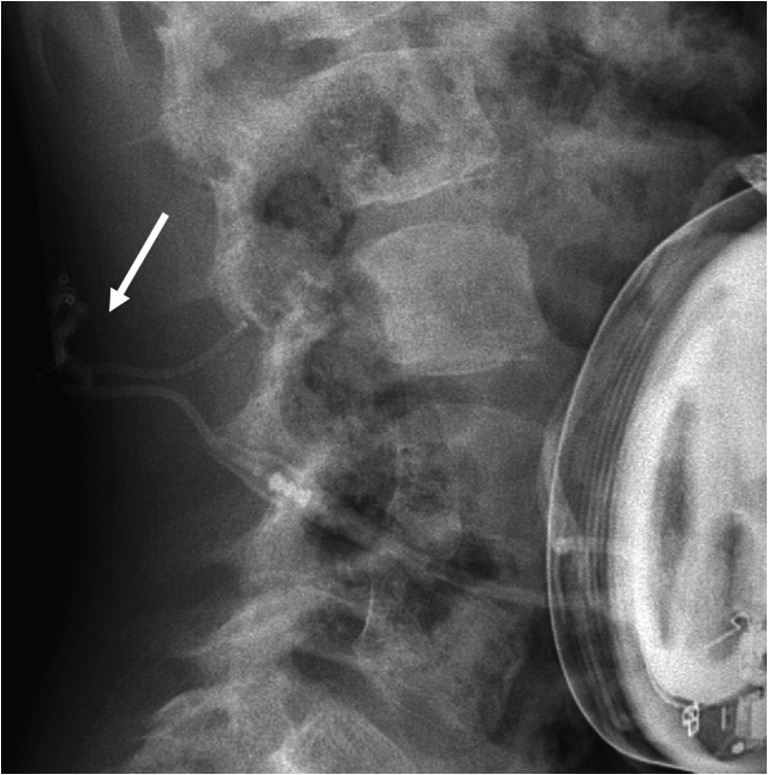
Case 4, lateral spine X-ray: the catheter is dislocated and a large part lies rolled up subcutaneously, so that it ends in the soft tissues between the lumbar spinous processes
